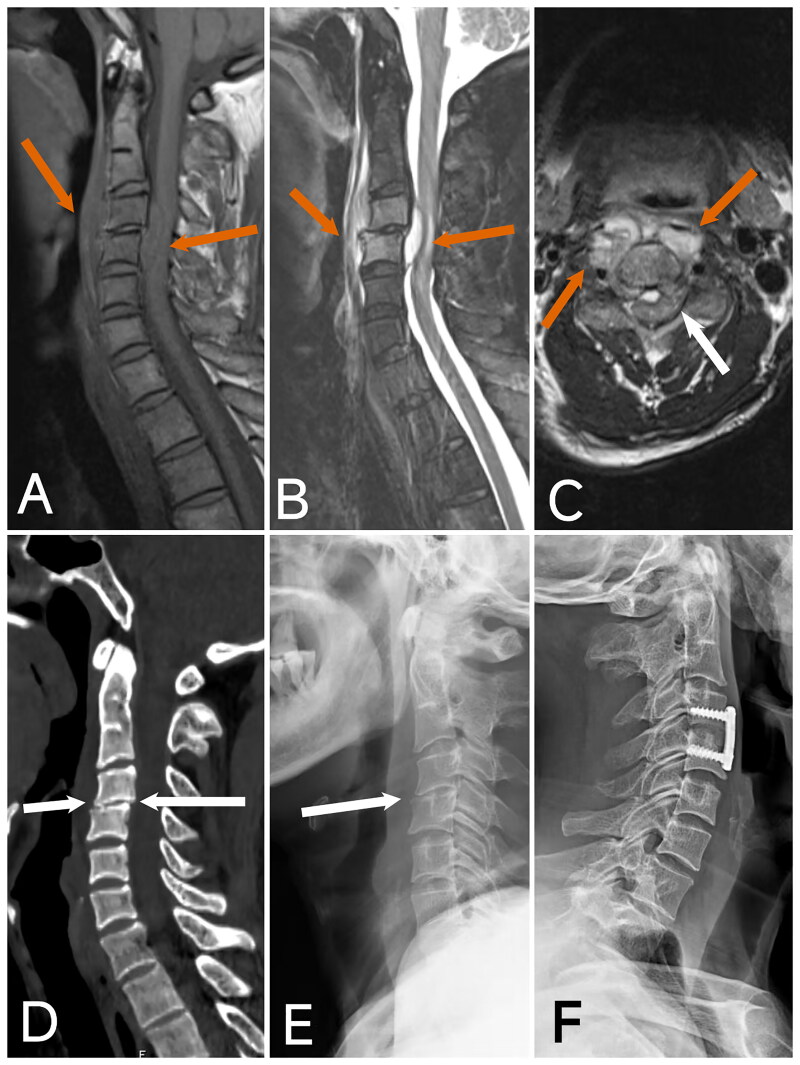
Image of case 2. A: T1-weighted imaging (T1WI); B: T2-weighted imaging with fat suppression (T2WI-FS); C: Axial T2WI shows a prevertebral abscess and epidural abscess with significant compression of the spinal cord, along with a linear area of high T1 and T2 signal within the spinal cord (red arrow). D, E: CT and X-ray reveal C2-3 segmental non-union, marked narrowing of the C4-5 intervertebral space, vertebral instability, slight posterior displacement of the C4 vertebra, and prevertebral bone destruction (white arrow). F: Postoperative X-ray at 4 months shows good positioning of the internal fixation, successful bone graft fusion, correction of the C4 posterior slip, restoration of cervical curvature, and recovery of intervertebral height.
